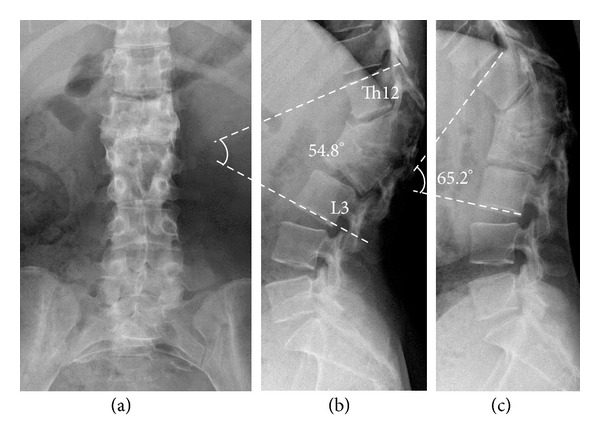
Presurgical plain radiographs: (a) AP view showing the bony fusion of the L1 and L2 vertebrae. (b) Lateral neutral view showing 54.8° of local kyphosis from T12 to L3. (c) Lateral flexion view showing worsening of the kyphosis to 65.2°.
